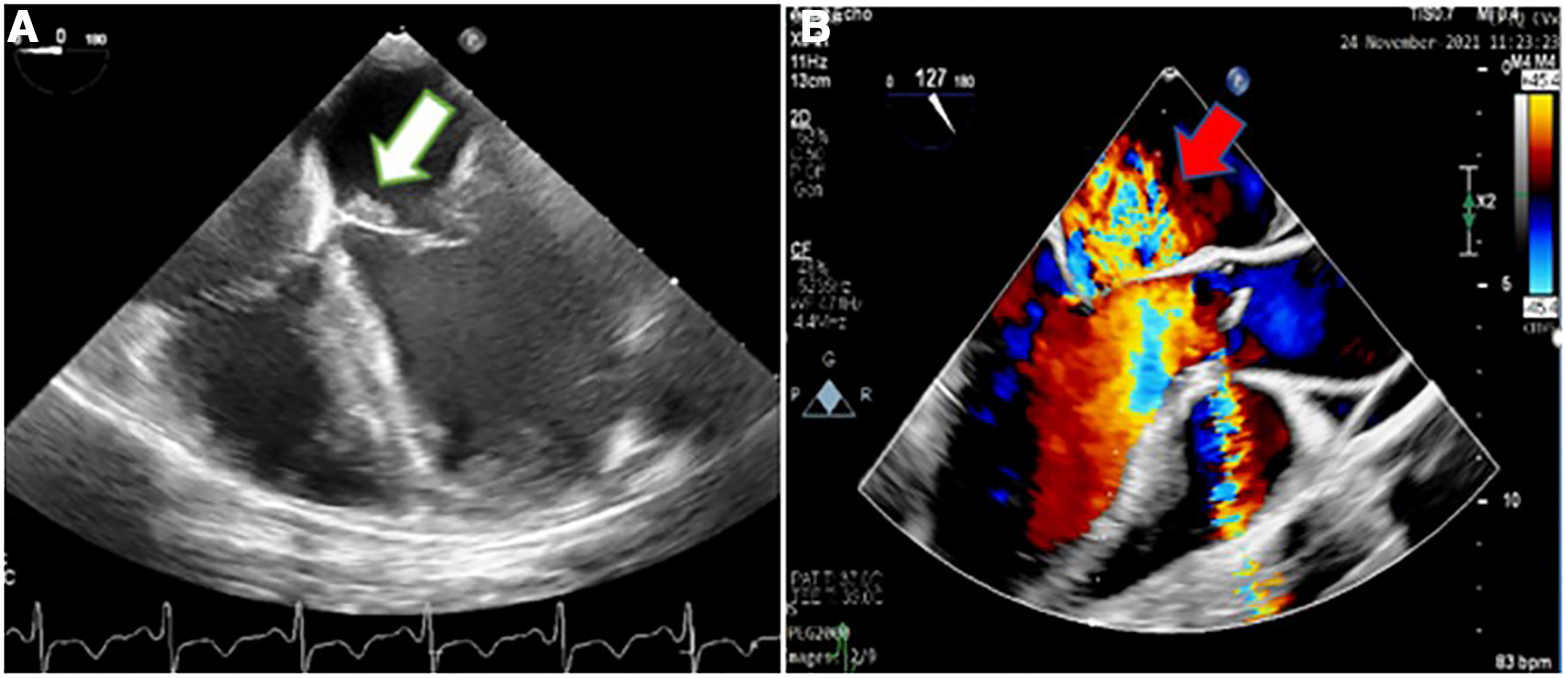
Two-dimensional transesophageal echocardiography showing an irregular thickening over the atrial side of the base of anterior mitral valve leaflet (white arrow) (A) suggestive of the involvement of the mitral valve by infective endocarditis, which caused severe mitral regurgitation, demonstrated in color Doppler flow study (red arrow) (B).
